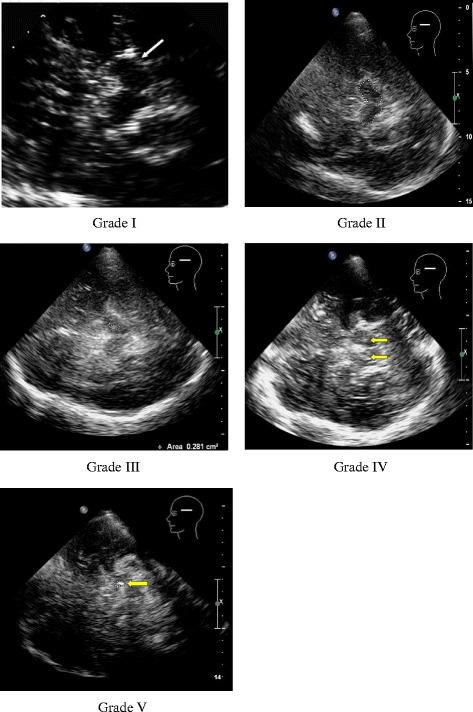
Grades of SN echogenicity by Transcranial ultrasound. Grade I: the same as brainstem; Grade II: with scattered points and thin lines slightly stronger than brainstem; Grade III: with patches of moderate echogenicity but weaker than brain pool; Grade IV: with patches of hyper echogenicity as the same as brain pool; Grade V: with patches of hyper echogenicity stronger than brain pool.
